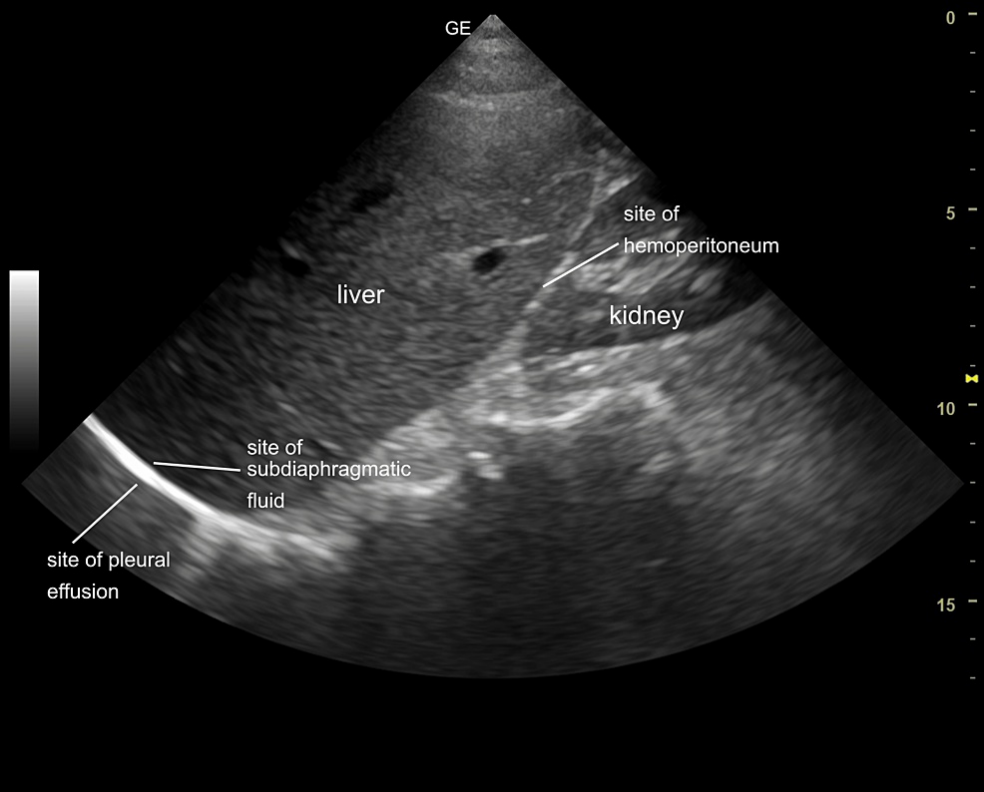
Right upper quadrant ultrasound image A typical ultrasound image was correlated with the gross anatomy and the sites of pleural, subdiaphragmatic, and hepatorenal fluid accumulation were identified.
